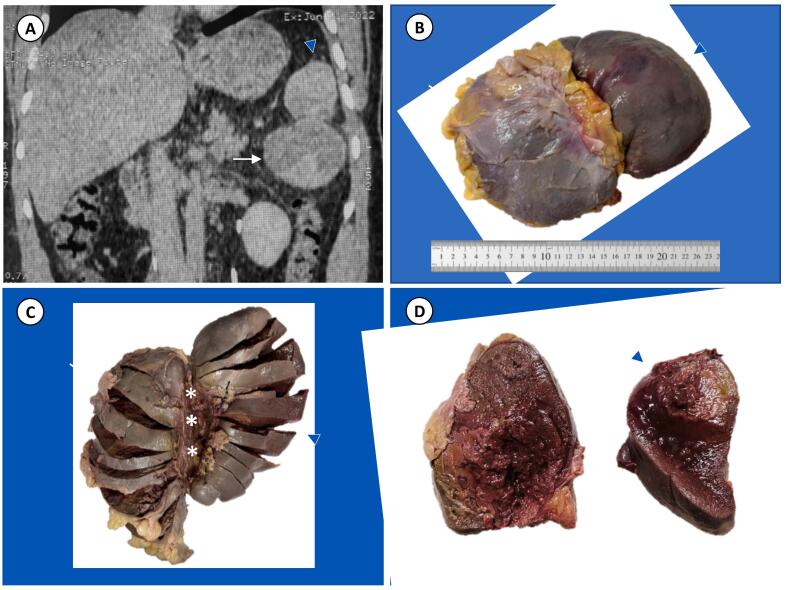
(Arrow; spleen, arrow head; accessory spleen). (A): Coronal reconstructed post-contrast CT images, showing the spleen in its normal position with homogeneous density and smooth outlines attached with another cystic heterogenous lesion with similar density at the lower pole of the spleen, compressing the left kidney. (B): Intact gross image of spleen attached to similar large mass. (C): The spleen and the AS are attached with each other by connective tissue (stars). (D): Cut surface of the spleen and accessory spleen exhibiting similar dark-red infarcted cut surface with persevered sub-capsular region. (For interpretation of the references to colour in this figure legend, the reader is referred to the web version of this article.)
